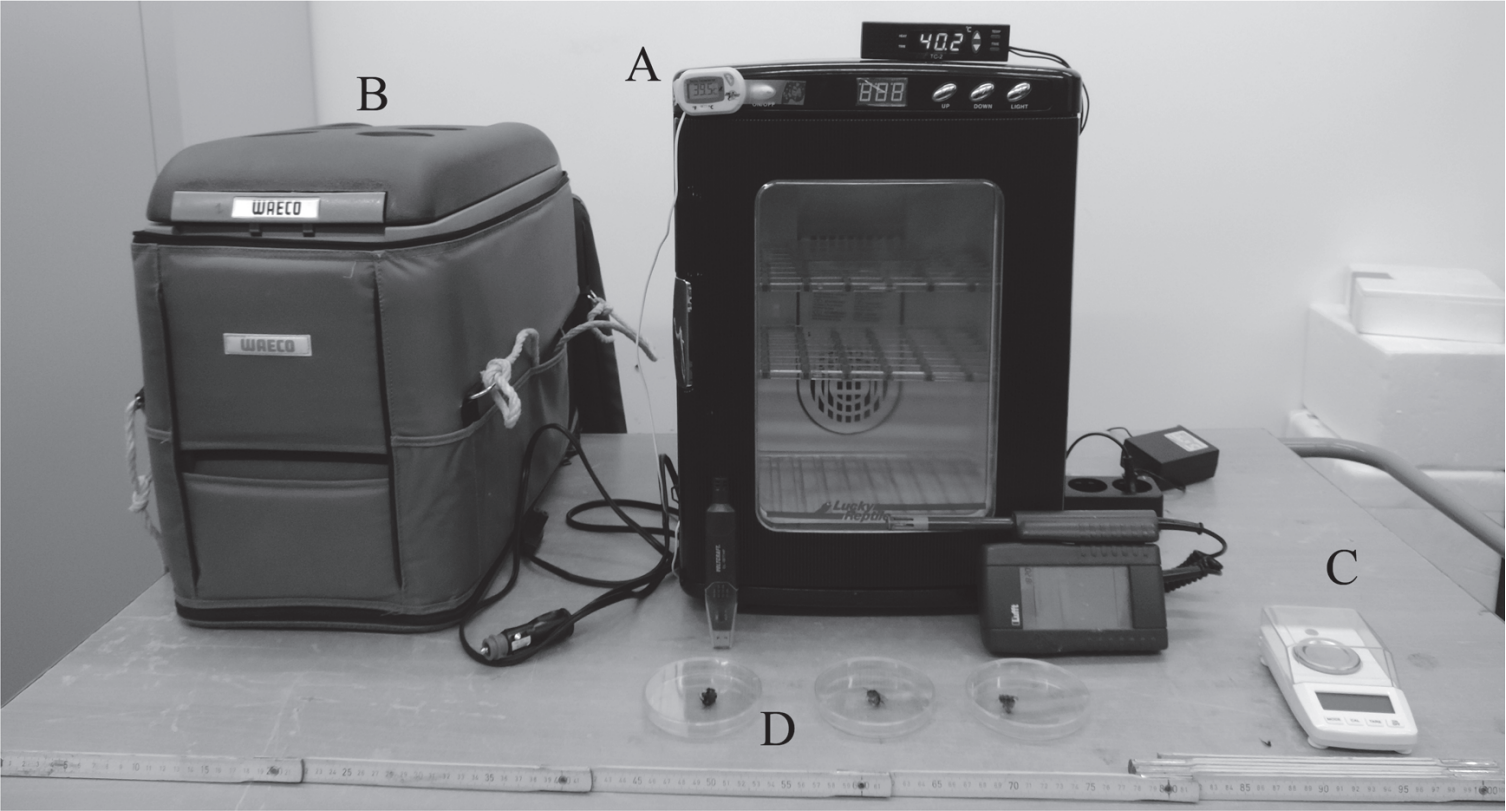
Picture of the experimental device. Picture of the experimental device: A) Incubator Herp Nursery II with its 12 V vehicle power supply, the datalogger (Voltcraft DL-181 THP USB Ambient Monitoring Data Logger), the psychrometer (Lufft C210), the digital thermometer (Zoo Med) and the thermostat (Lucky Reptil ThermoControl Pro II) B) Fridge WAECO CDF to keep bumblebees at 8°C with 12 V vehicle power supply, C) Scale Ace CT-50, D) Petri Dishes with tested bumblebees.
